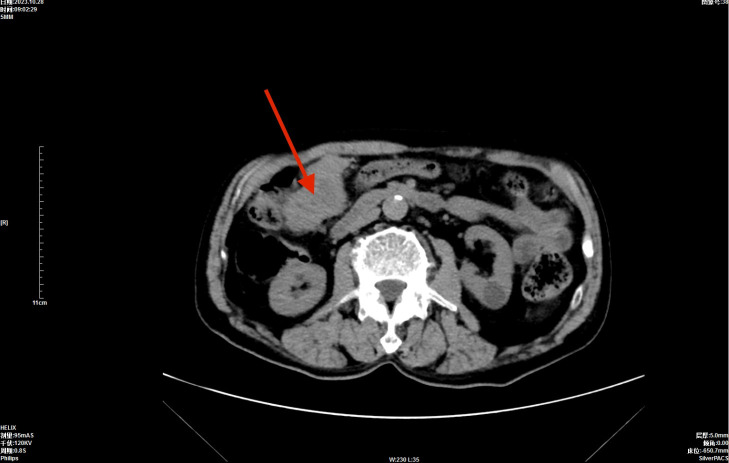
Enhanced CT showing an irregular mass shadow (arrow) in the right mid-upper abdomen.
